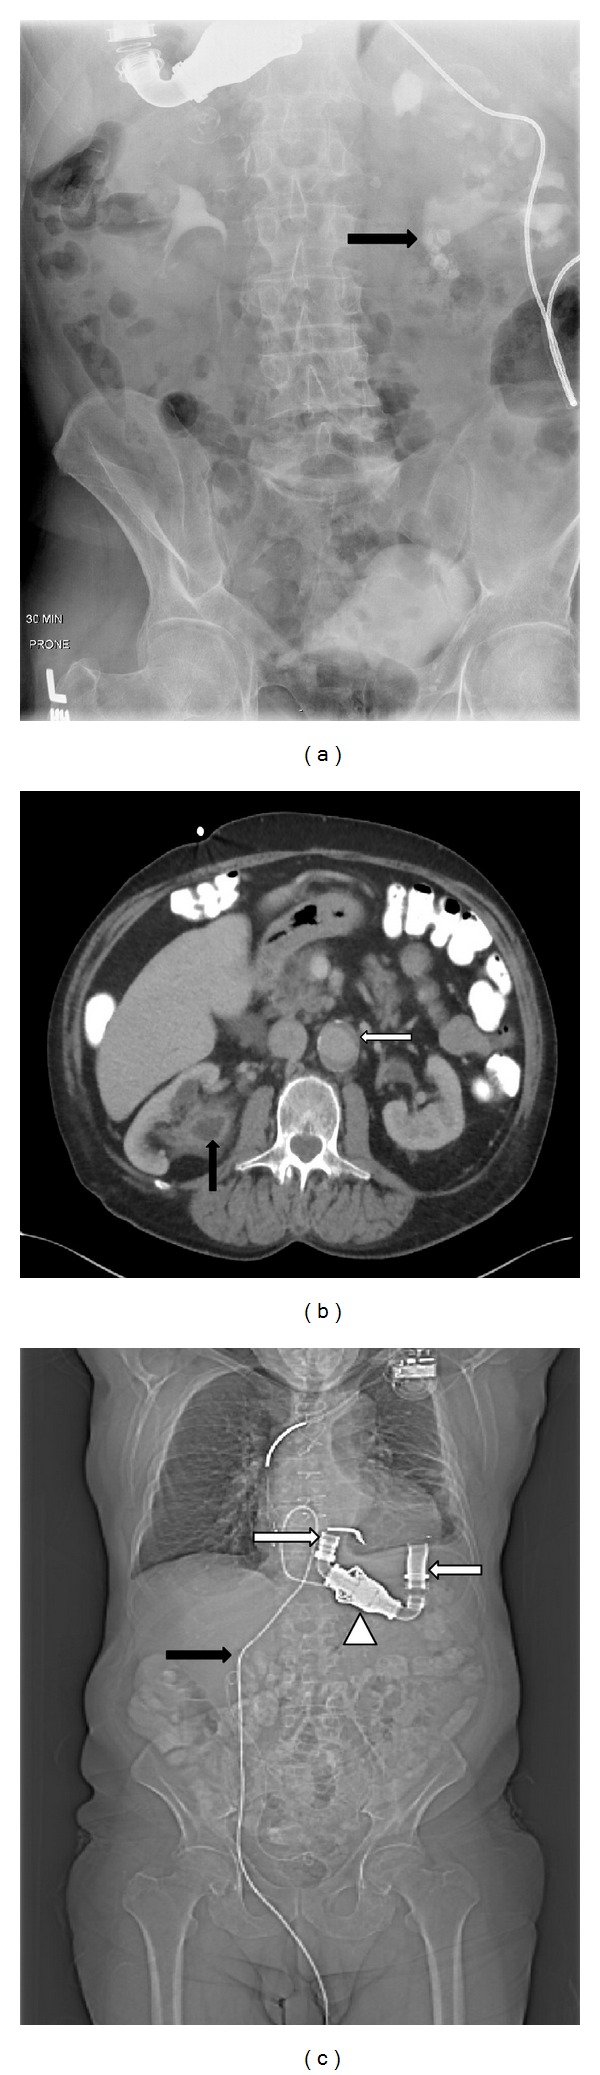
(a) Intravenous pyelogram image showing multiple right renal pelvis stones (black arrow). (b) Axial CT image at the level of the renal hilum showing incidental aortic aneurysm (white arrow) and right renal pelvis filling defect representing urothelial carcinoma (black arrow), and (c) scout CT image showing the location of LVAD cannulae (white arrows), continuous flow pump (white arrow head), and percutaneous connection cord (black arrow).
